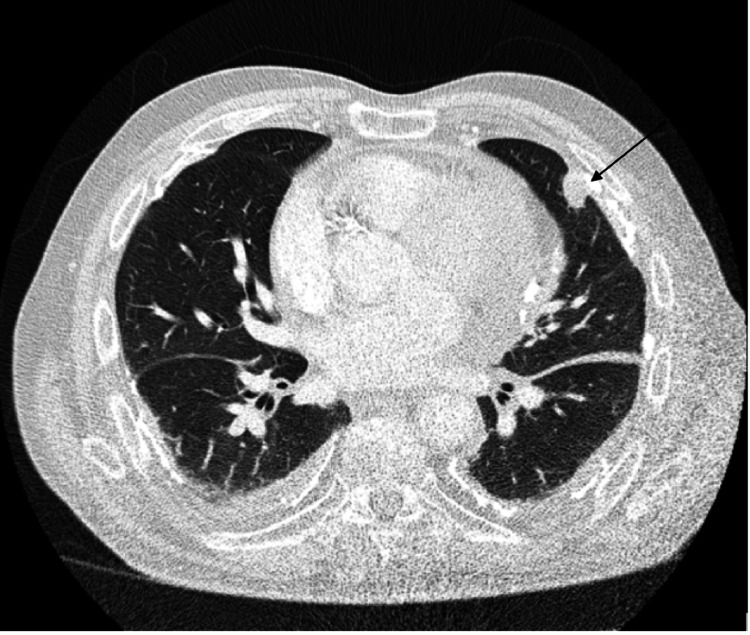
Axial slice CT scan showing lingular nodule that provided tissue diagnosis
